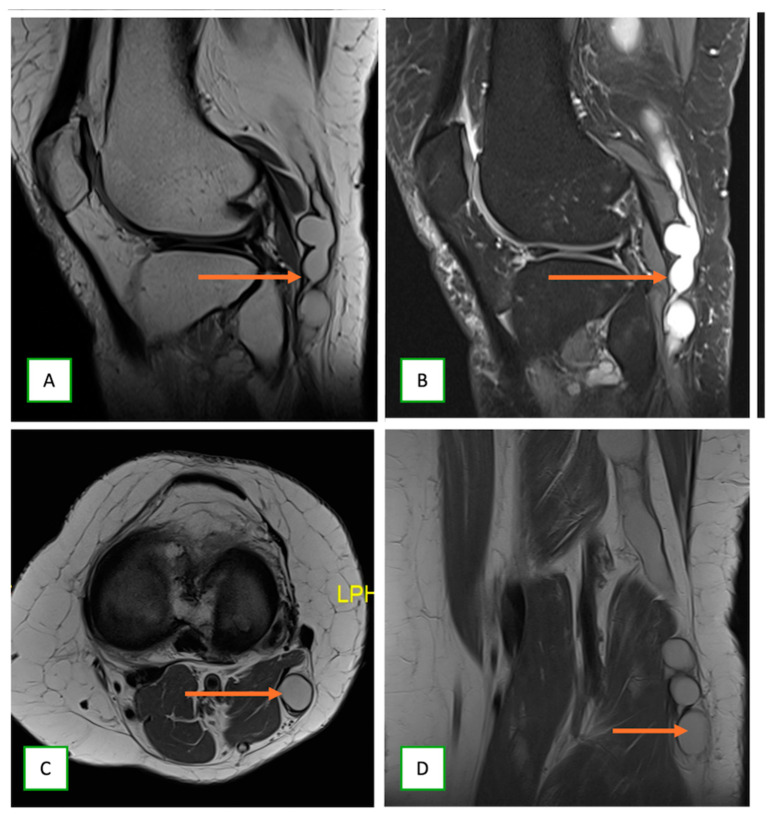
Intraneural ganglion cyst of the tibial nerve in a 39-year-old woman with a painful lump behind the left knee. Sagittal T1 (A), sagittal fat-saturated T2 (B), axial T1 (C), and coronal T1 (D) images show the cystic lesion within the nerve (thin arrow), which appears low on T1- and high on T2-weighted images.
